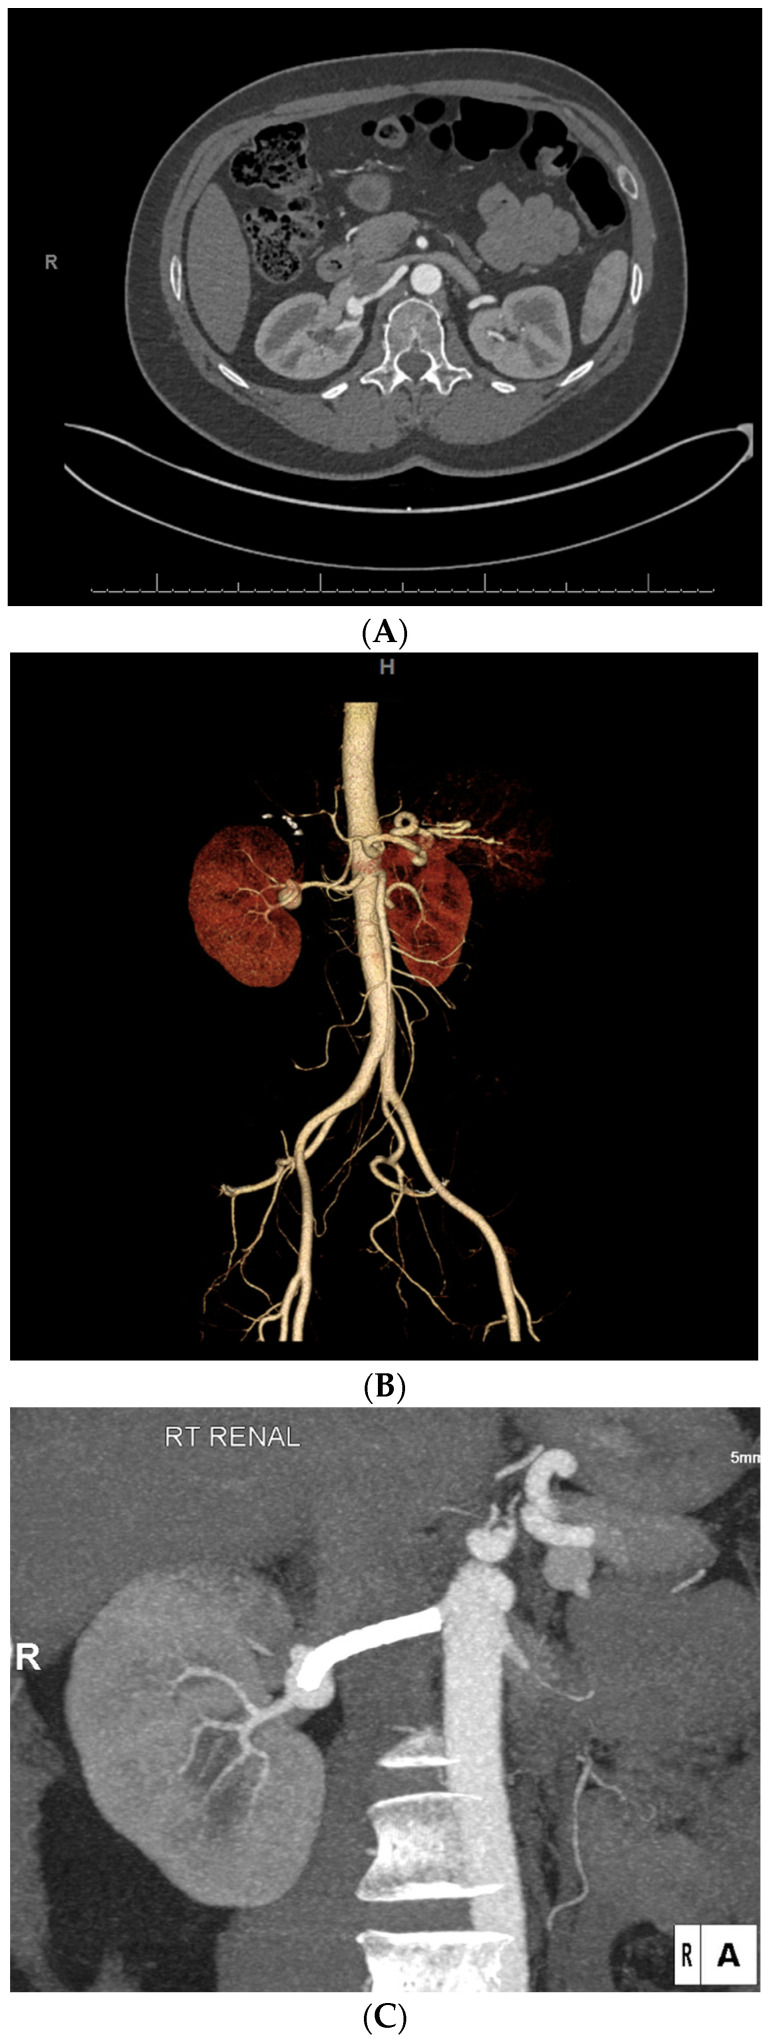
A 57-year-old woman with a significant medical history including gastroparesis, eosinophilic esophagitis with prior dilation in 2020, type 2 diabetes mellitus, multiple sclerosis, anxiety, postoperative nausea and vomiting triggered by narcotics, and multiple medication allergies. She sought evaluation due to an 18 mm right renal aneurysm discovered during a recent CT scan prompted by right flank pain. After consultation, she opted to undergo the recommended surgical intervention for the aneurysm. The computed tomographic angiography of the abdomen and pelvis, both without and with contrast, revealed a saccular aneurysm located at the bifurcation of the right renal artery. The neck size of the aneurysm measures 14 mm, with a maximal diameter of approximately 1.8 cm in the axial plane. After the procedure, follow-up imaging revealed a new stent extending along most of the right renal artery, spanning across an aneurysm located at the renal hilum, which is positioned at a trifurcation. The stent is patent, and the aneurysm remains perfused, measuring approximately 1.7 × 1.5 cm, unchanged from the previous examination (A–C).
